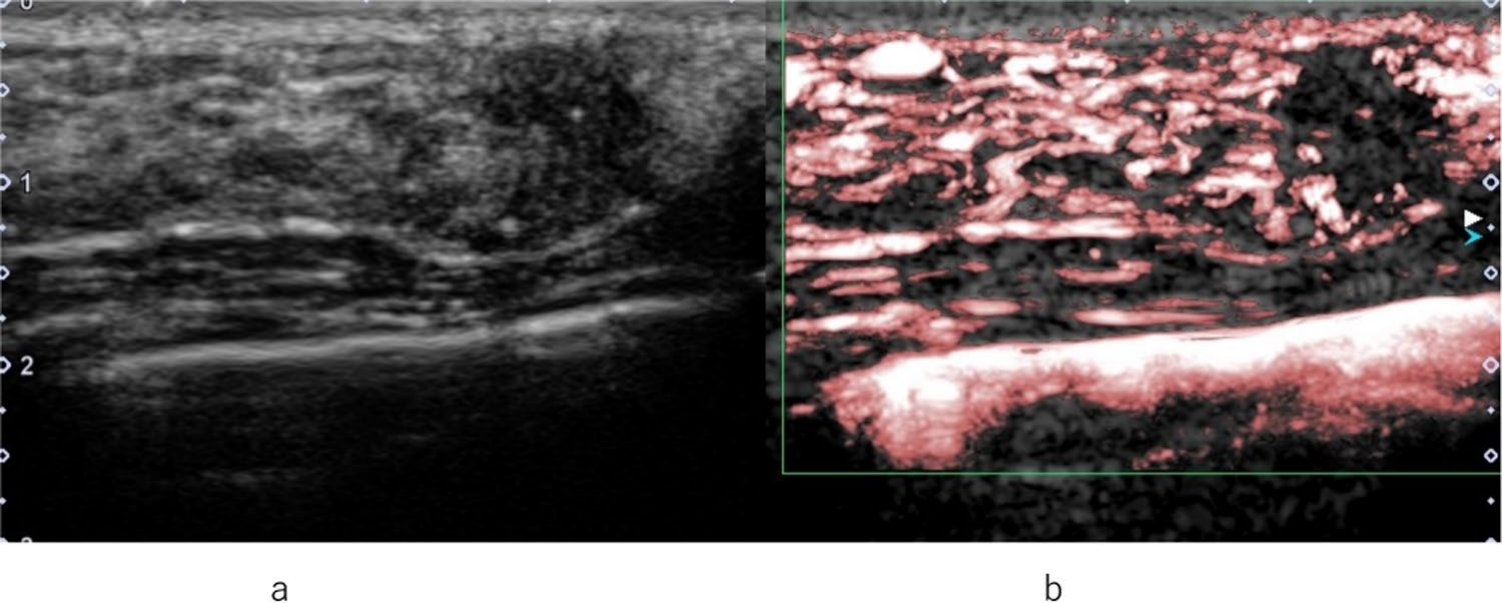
Ductal carcinoma in situ. a Conventional ultrasound shows a hypoechoic area in the mammary gland with echogenic foci. b Contrast-enhanced ultrasound shows heterogeneous enhancement with a clear defect
